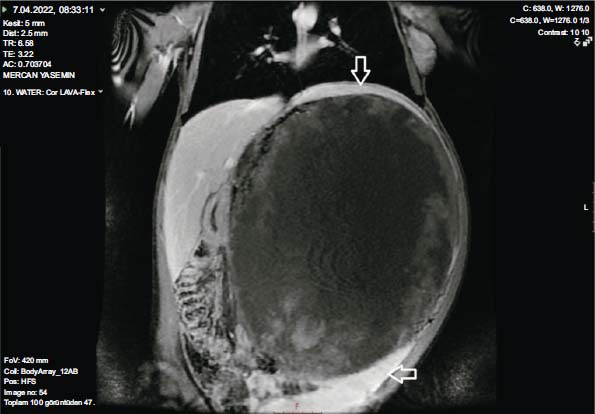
Complex cystic mass with solid (gaucheroma) areas filling the left half of the abdomen in the spleen site on T1-weighted contrast-enhanced coronal MR imaging. Parts of intact spleen tissue were also observed in the upper and lower poles.
